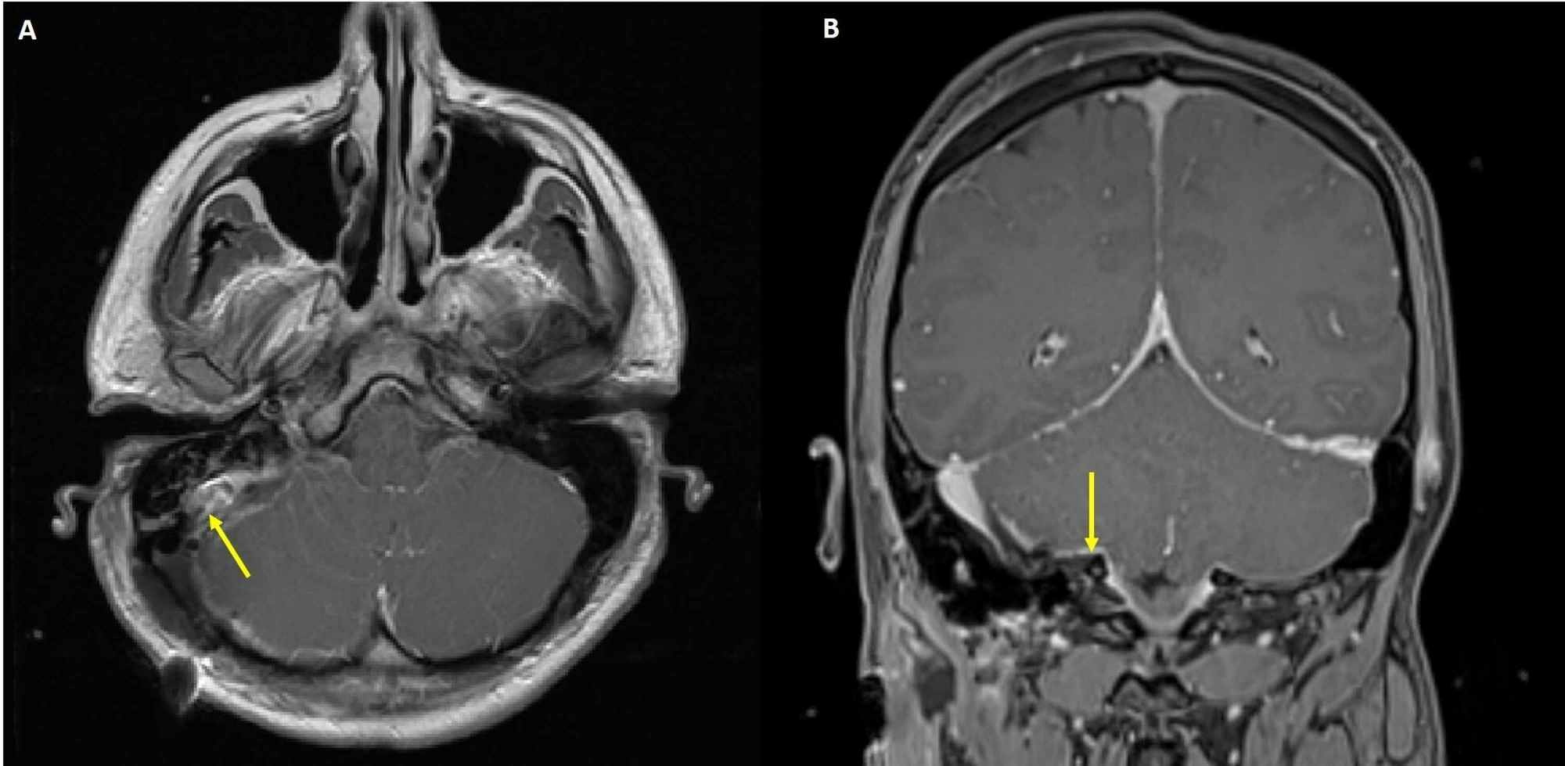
Post-operative magnetic resonance imaging (MRI) brain with gadolinium contrast (A) Axial and (B) coronal views showing partial resection of tumor at the right sigmoid sinus region (solid yellow arrows)
